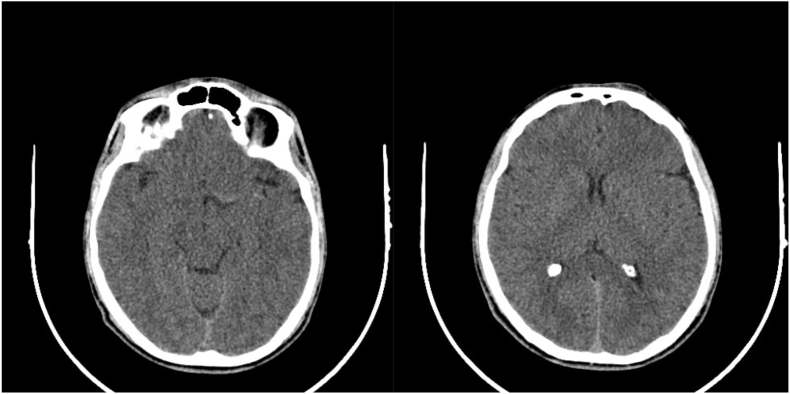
A and B showing normal head CT scans.
